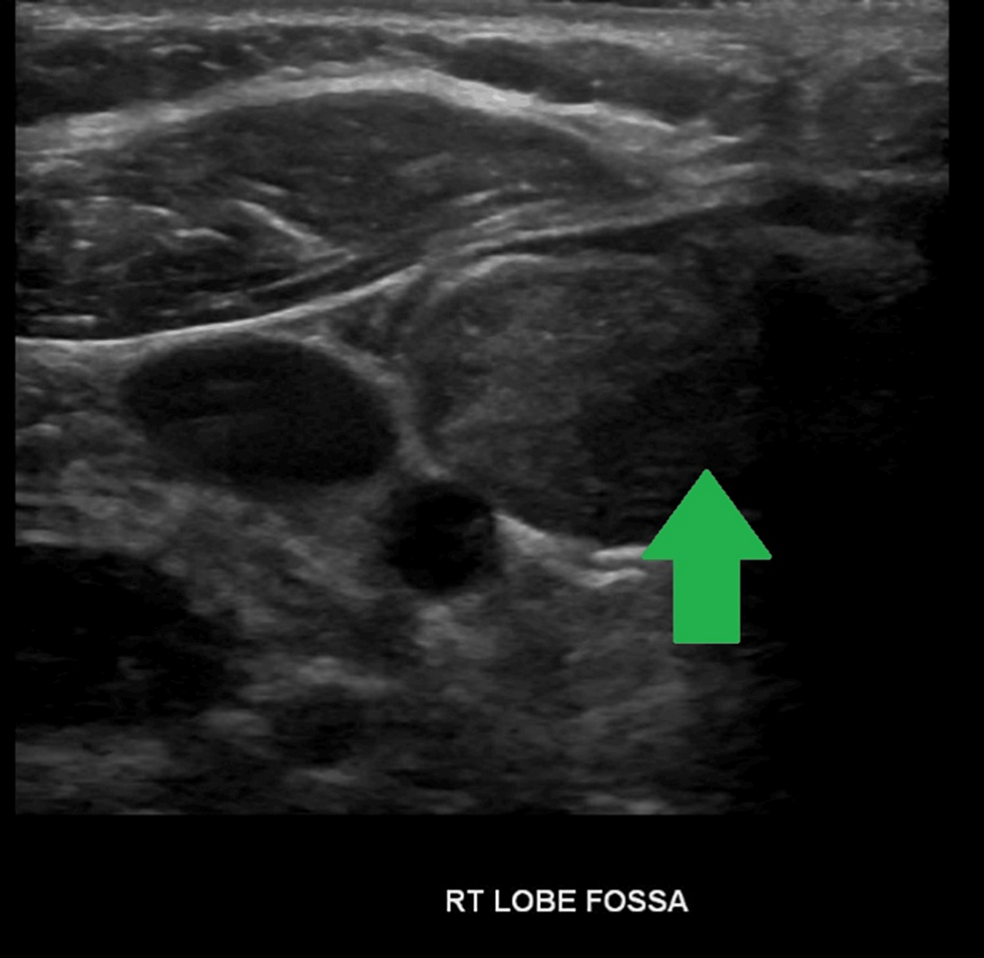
Neck ultrasound showing regrowth of thyroid tissue
